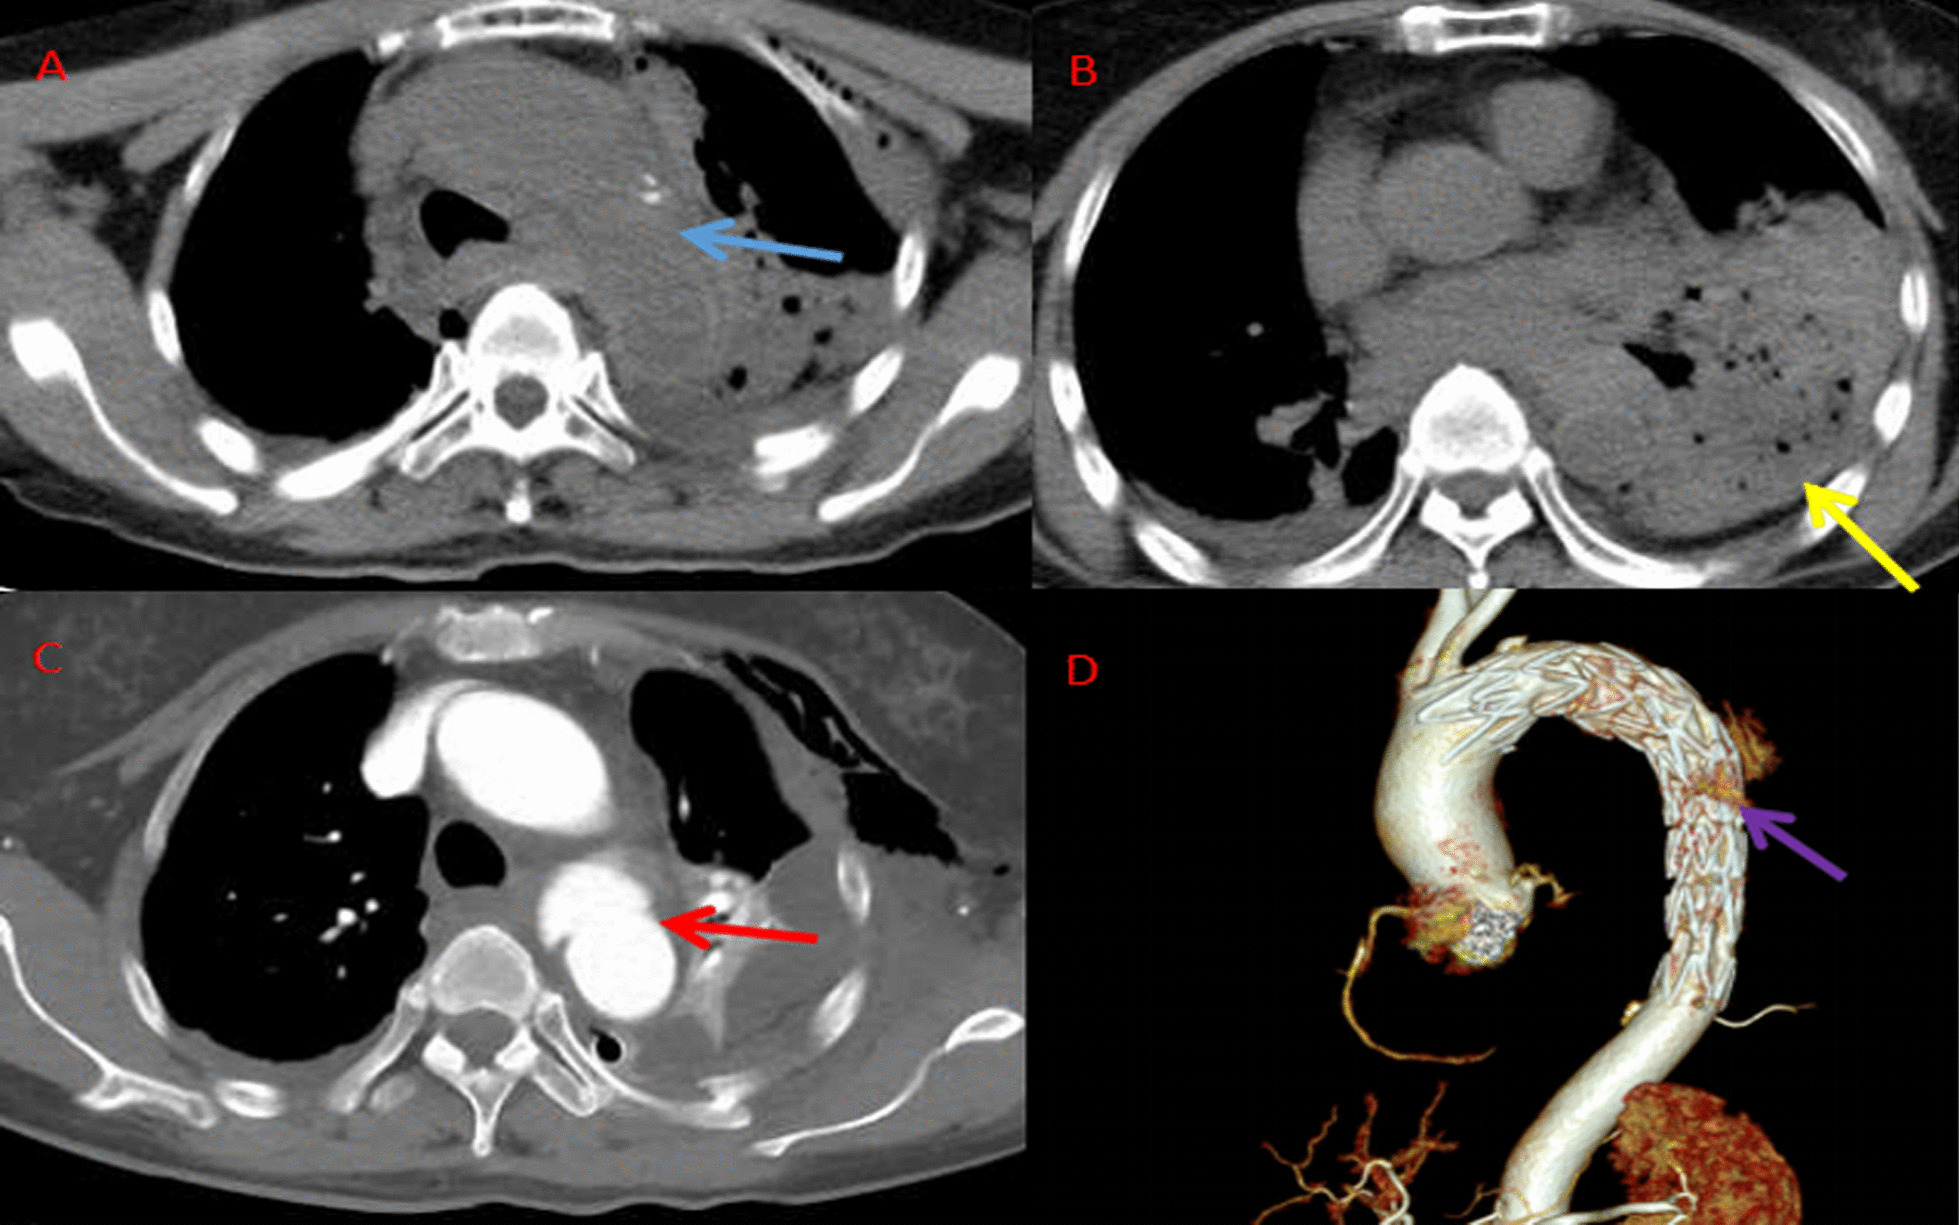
Imaging scan of Case 1. A, B A plain CT scan of the chest indicated a blurred mediastinal space with effusion (blue arrow), gastric hernia in the thoracic cavity (yellow arrow), and mediastinal gas and effusion/hemorrhage, and left diaphragmatic hernia was considered. C, D Vascular CTA indicated a possibility of dissection of the descending aorta (red arrow, C), and femoral artery stent implantation with a thin mesh was performed (purple arrow, D)
